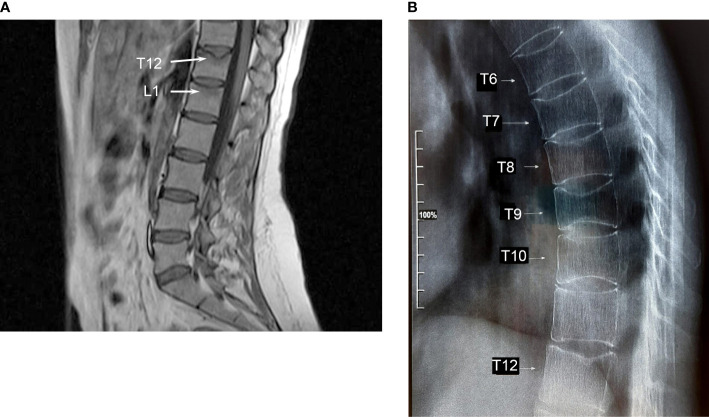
(A) Magnetic resonance imaging of the lumbar spine revealing the presence of vertebral fractures of T12 and L1. Peri-lesion edema of both vertebrae indicating recent fractures is shown (arrows). (B) X-ray of the thoracic spine shows vertebral fractures from T6 to T9.
